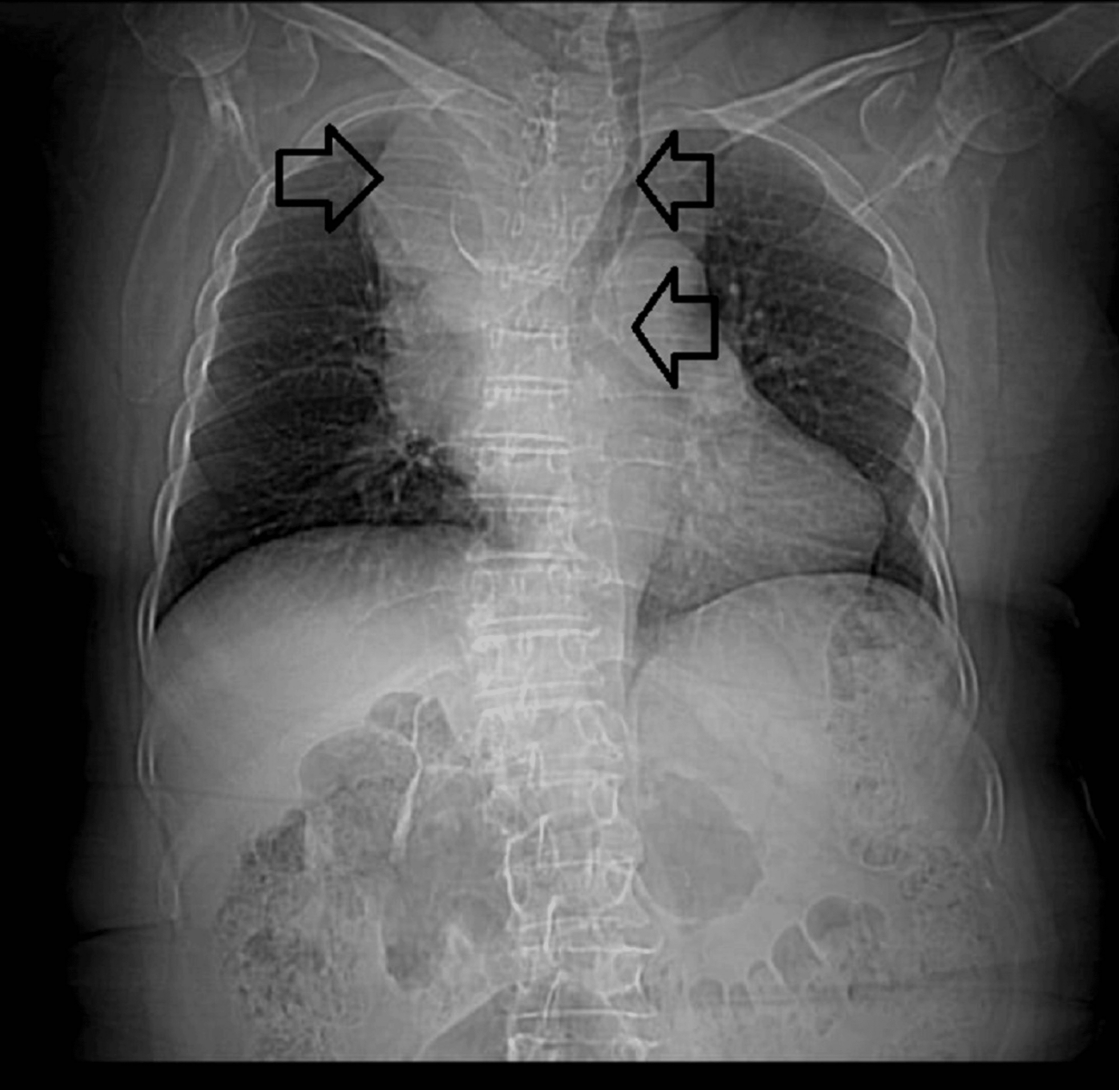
Non-contrast chest CT (coronal view) showing a bilobulated mass located in the anterior–superior mediastinum, causing compression of the superior vena cava and the brachiocephalic trunk, with rightward displacement of mediastinal structures and associated opacification, consistent with chronic superior vena cava obstruction
